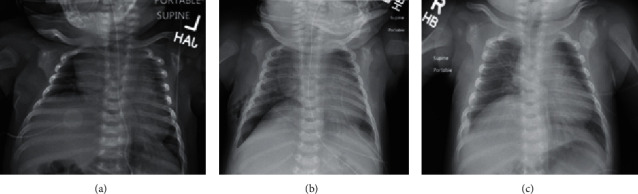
(a) Chest radiograph prior to surgery showing elevation of right hemidiaphragm. (b) Chest radiograph immediately after surgery demonstrating expansion of the right lung field with small basilar pneumothorax and minimal subcutaneous emphysema. (c) Chest radiograph one-month postoperatively demonstrating continued increased right hemithorax volumes with excellent lung expansion.
